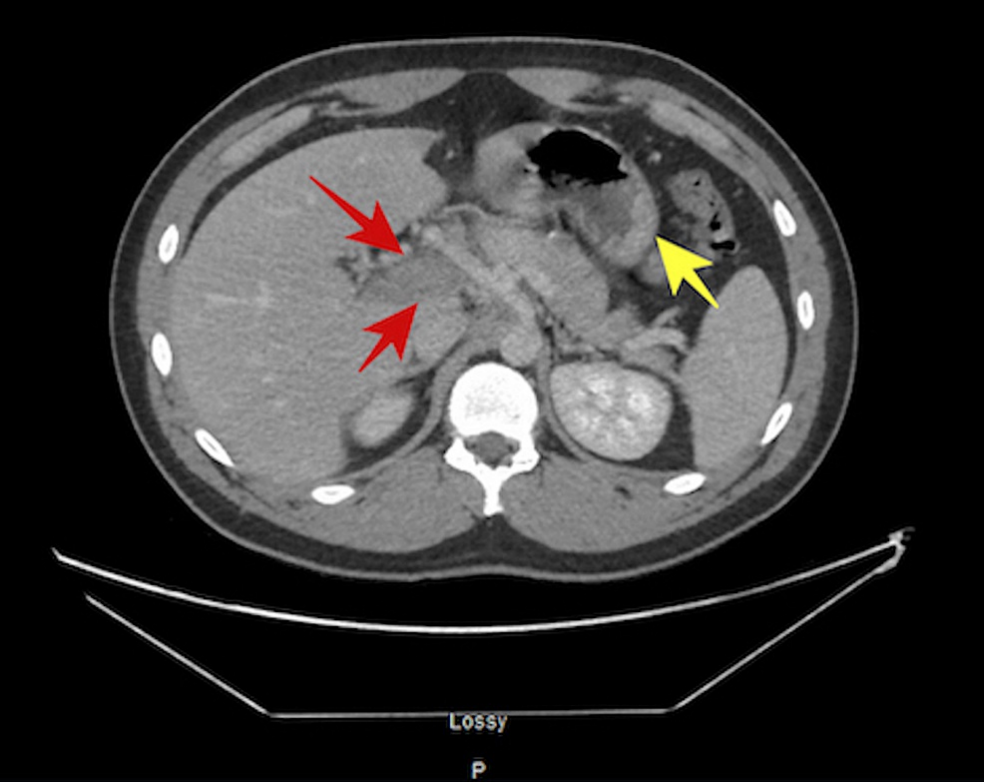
Axial CT scan of the abdomen shows an area of low attenuation in the region of portal vein suggestive of portal vein thrombosis (red arrows) and submucosal fat attenuation and signs of inflammation in the visualized portion of the colon suggestive of burned-out colitis or inflammatory bowel disease (yellow arrow).
